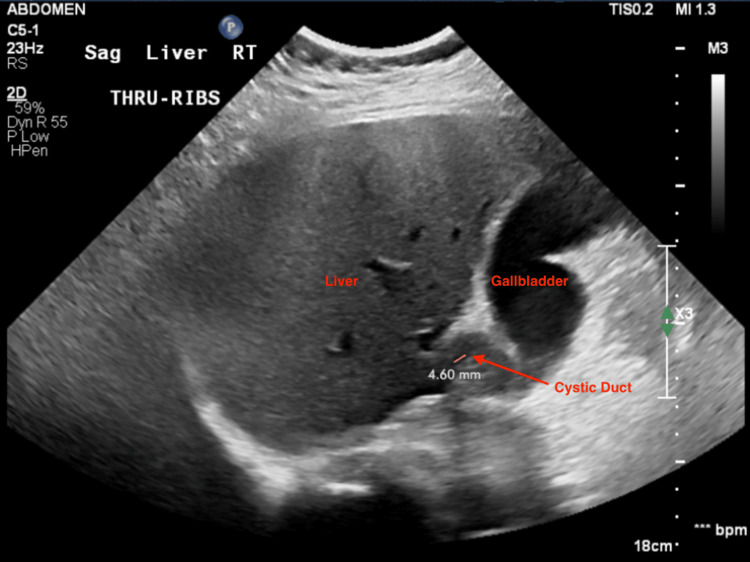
Right upper quadrant ultrasound, displaying the short cystic duct (indicated by a red arrow)
